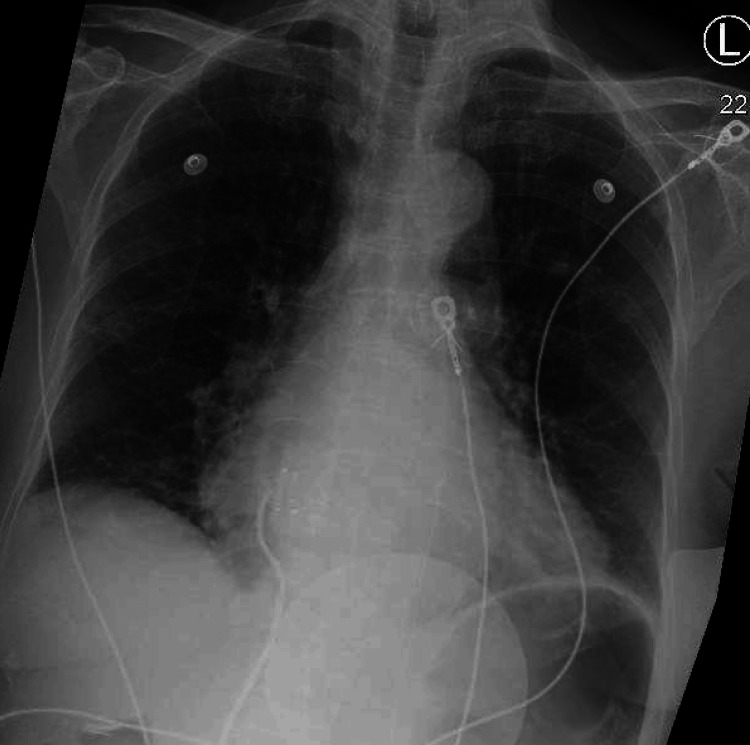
A chest roentgenogram on admission shows prominent upper zone vessels, an enlarged cardiac silhouette, and aortic arch calcifications
